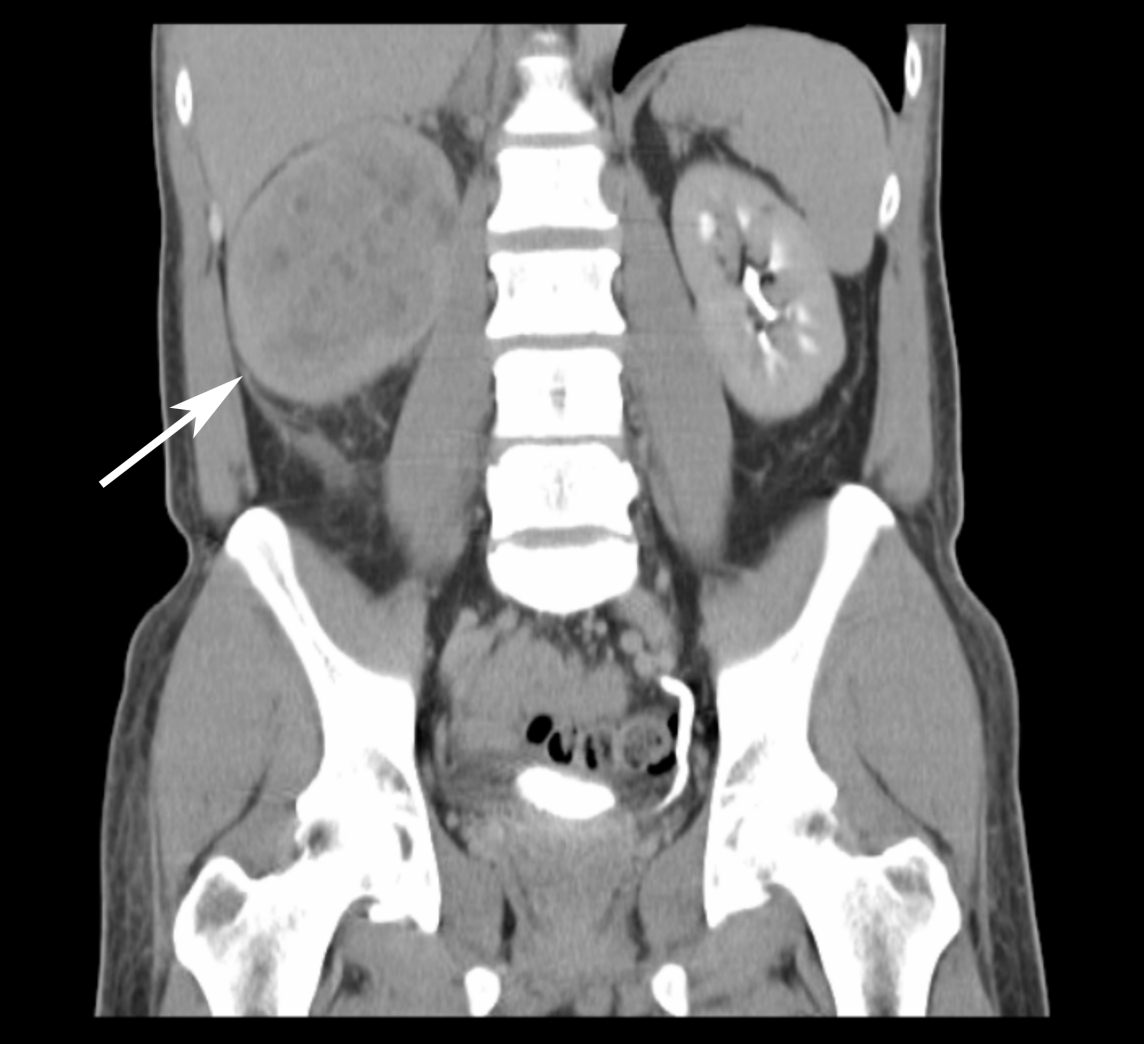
Computed tomography (CT) coronal view of our patient's thorax and abdomen, showing a large right renal cell carcinoma (arrow) 4 months later. This image is in the same coronal cut as Figure 1, as can be seen from evaluation of the vertebral column.
